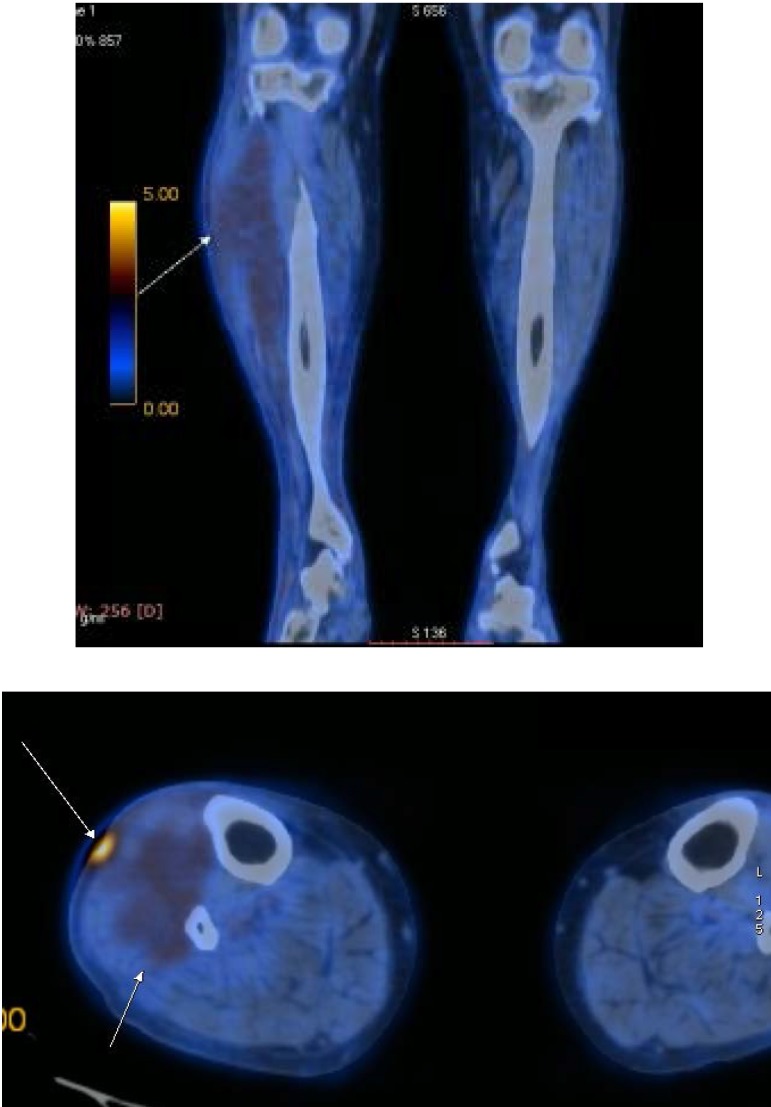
Increased intake of 18-FDG on the external compartment of the right calf (Personal photographic archive)
